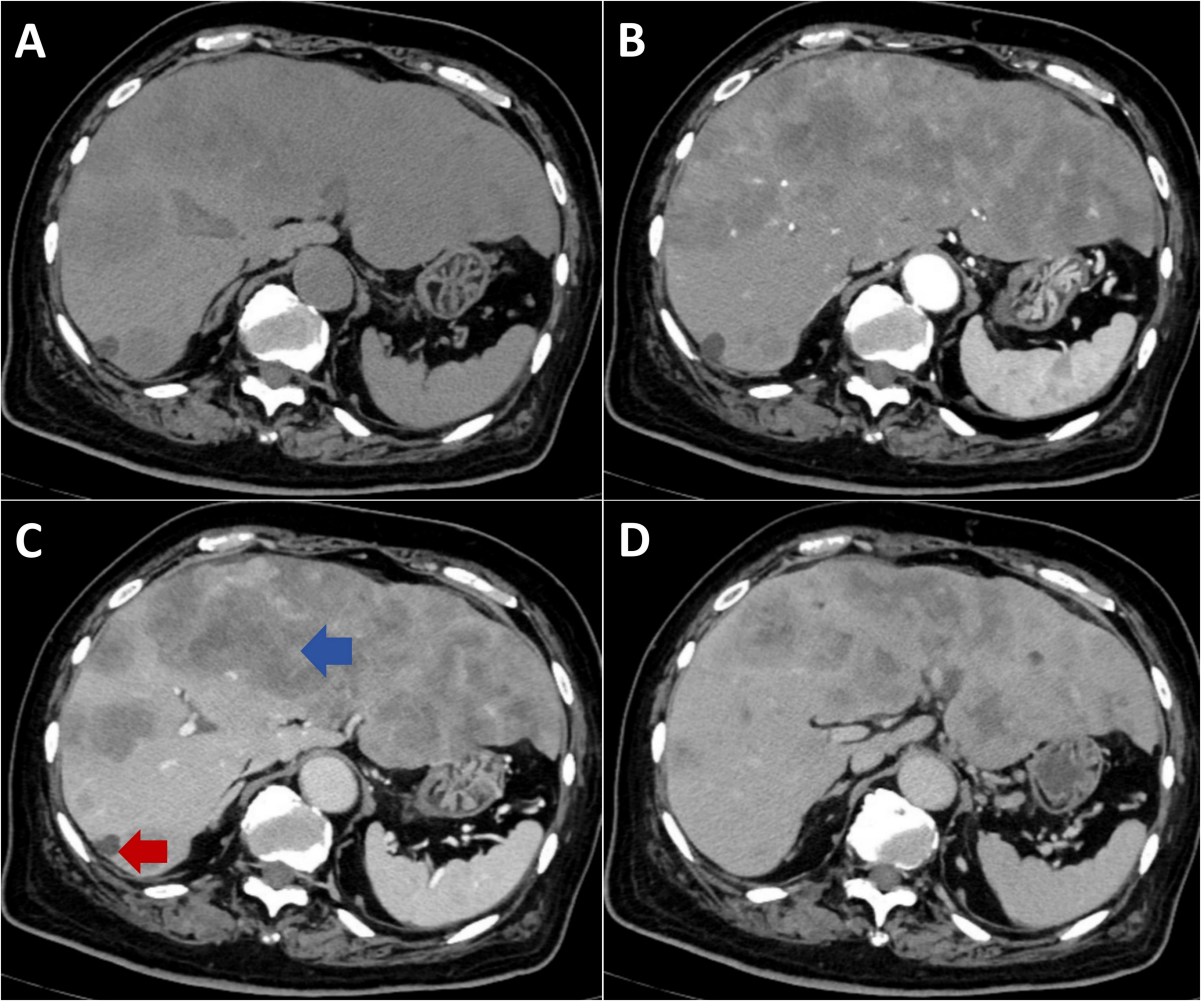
Contrast-enhanced CT imaging of metastatic hepatic lesions. (A) Non-contrast phase shows multiple hypodense lesions with ill-defined margins and coalescence throughout the liver. (B) Arterial phase demonstrates irregular peripheral rim enhancement with central hypoattenuation. (C) Portal venous phase reveals progressive peripheral enhancement. (D) Delayed phase shows sustained rim enhancement with minimal central fill-in.
